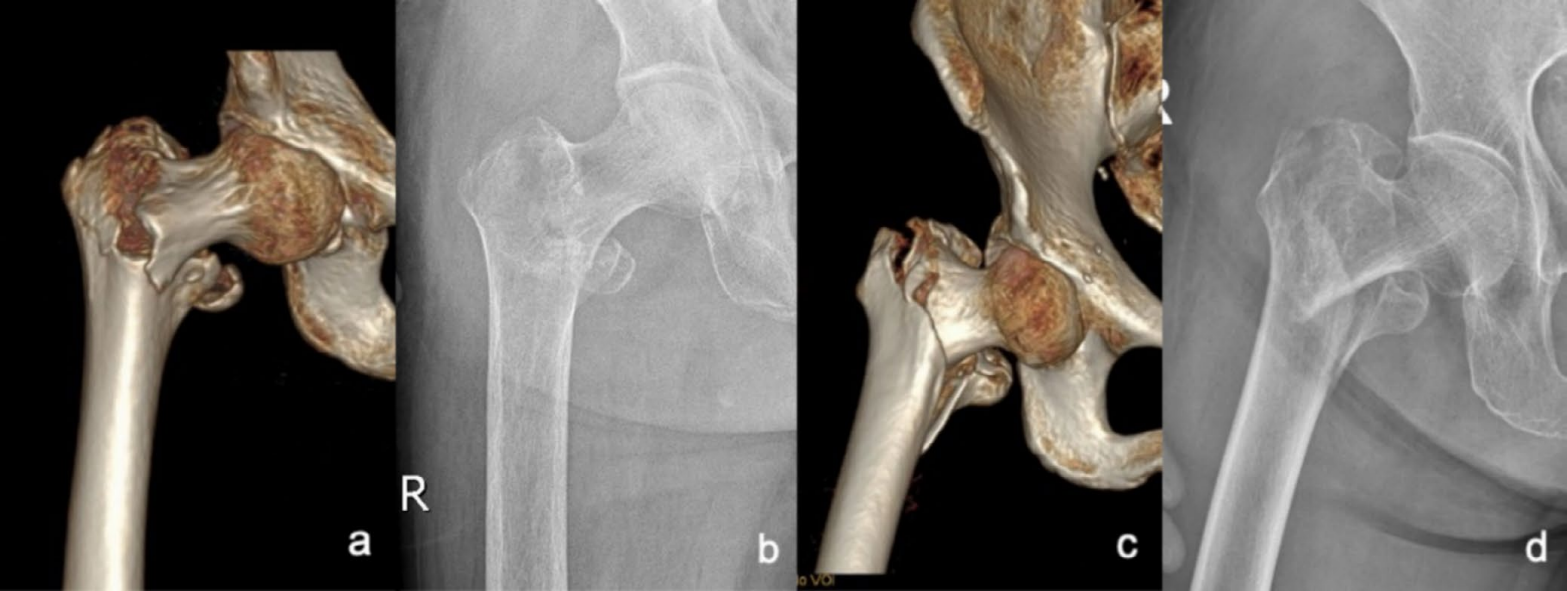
Comparison of preoperative images of irreducible type II‐1 fractures and reducible type I fractures. An irreducible type II‐1 fracture and a reducible type I fracture appear significantly different in 3D reconstructed CT images (a and c respectively) but are difficult to distinguish in anteroposterior X‐ray images (b and d, respectively).
